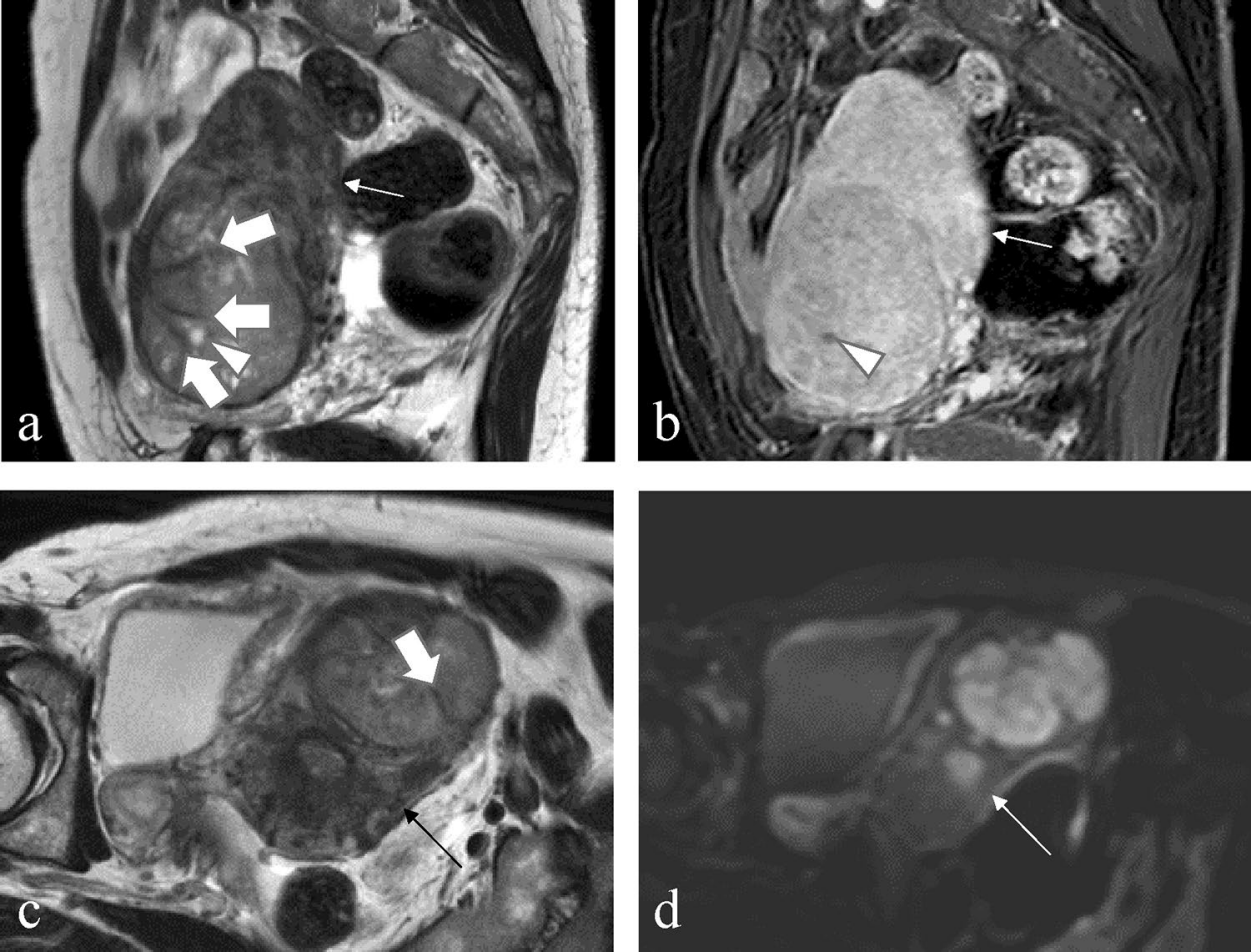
A 48-year-old woman with endometrial stromal sarcoma without abnormal vaginal bleeding. a The sagittal T2-weighted image and b the sagittal contrast-enhanced T1-weighted image with fat suppression reveals a tumor, locates mainly in the myometrium, with cyst (arrowhead). a The sagittal T2-weighted image and c the axial T2-weighted image indicates the lesion with T2-hypointense bands (arrow). d On an axial diffusion-weighted image with a *b*-value of 1000 s/mm^2^, the tumor showed a higher signal than the myometrium (thin arrow). The lesion shows restricted diffusion, and the mean apparent diffusion coefficient value is 1.03 × 10^−3^ mm^2^/s
